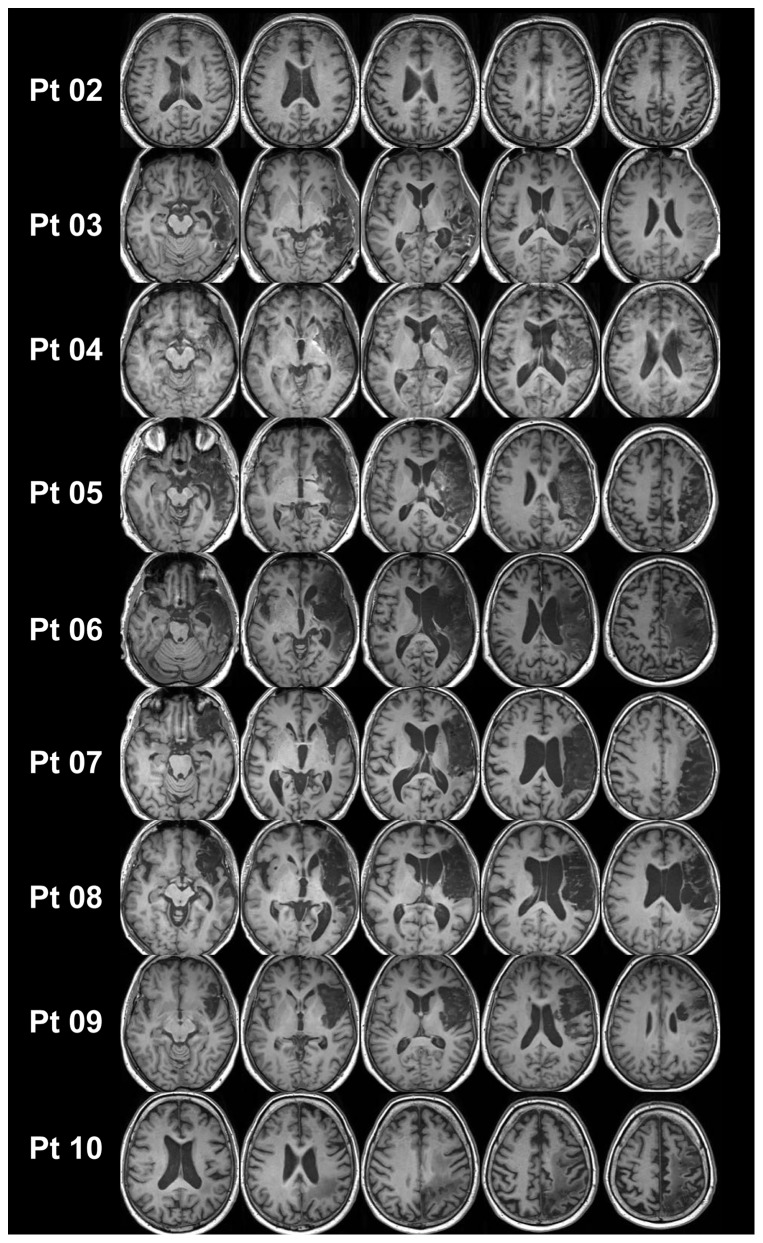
Lesion patterns of individual patients. Patient numbers correspond to patient IDs in Table 1 (patients #2-10). No MRI was available for patient #1. Right side of the brain corresponds to right hemisphere.
